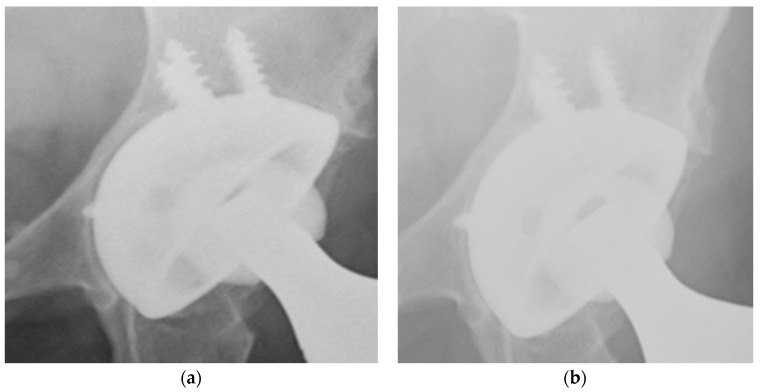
X-rays show good osteointegration of the implant and formation in a 72-year-old woman treated with cup revision for a Paprosky 3a. (a) One month follow-up, presence of radiolucent lines, (b) One year follow-up, shows filling of the radiolucent line with the formation of new bone.
